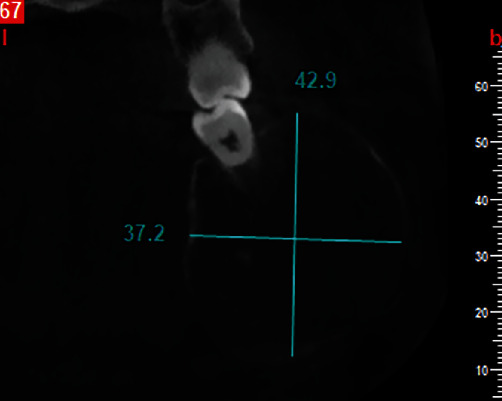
Labiolingual and superoinferior size of the lesion on cross-sectional CBCT section. (labiolingual size = 37.2 mm, superoinferior size = 42.9 mm).
